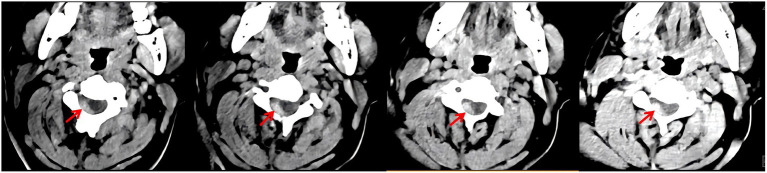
Cervical spine CT. A slightly hyperdense mass is observed in the right posterolateral epidural space (red arrows), consistent with an acute epidural hematoma.
